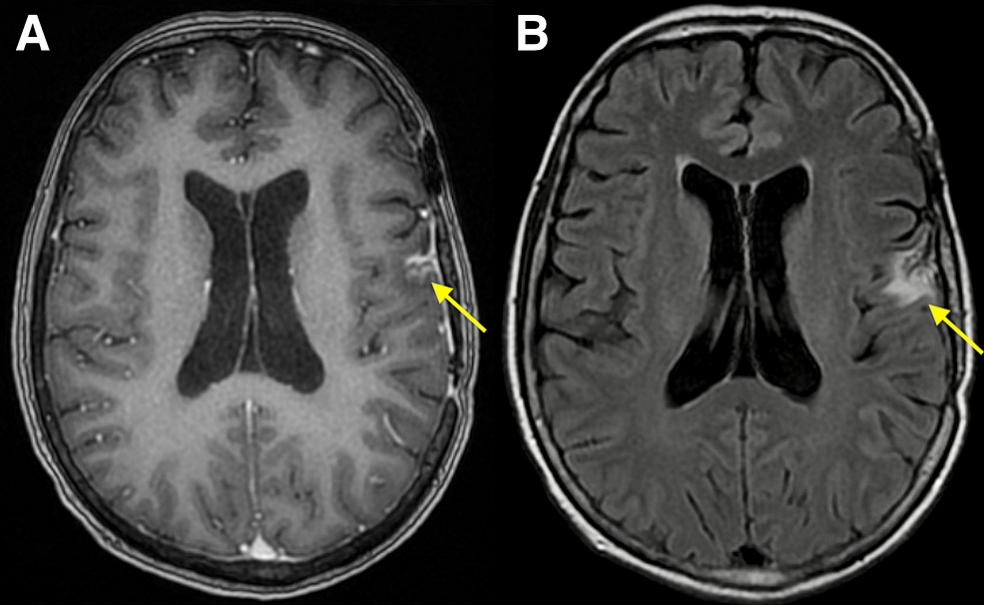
MRI 40 months after Gamma Knife stereotactic radiosurgery, 8 months after resection. Axial contrast-enhanced T1-weighted (A) and T2-weighted FLAIR brain MRI (B) obtained eight months after resection of cavernous malformation demonstrating stable postsurgical changes status post left frontal craniotomy with unchanged minimal enhancement at the resection cavity site and mild surrounding edema. FLAIR: Fluid-attenuated inversion recovery
